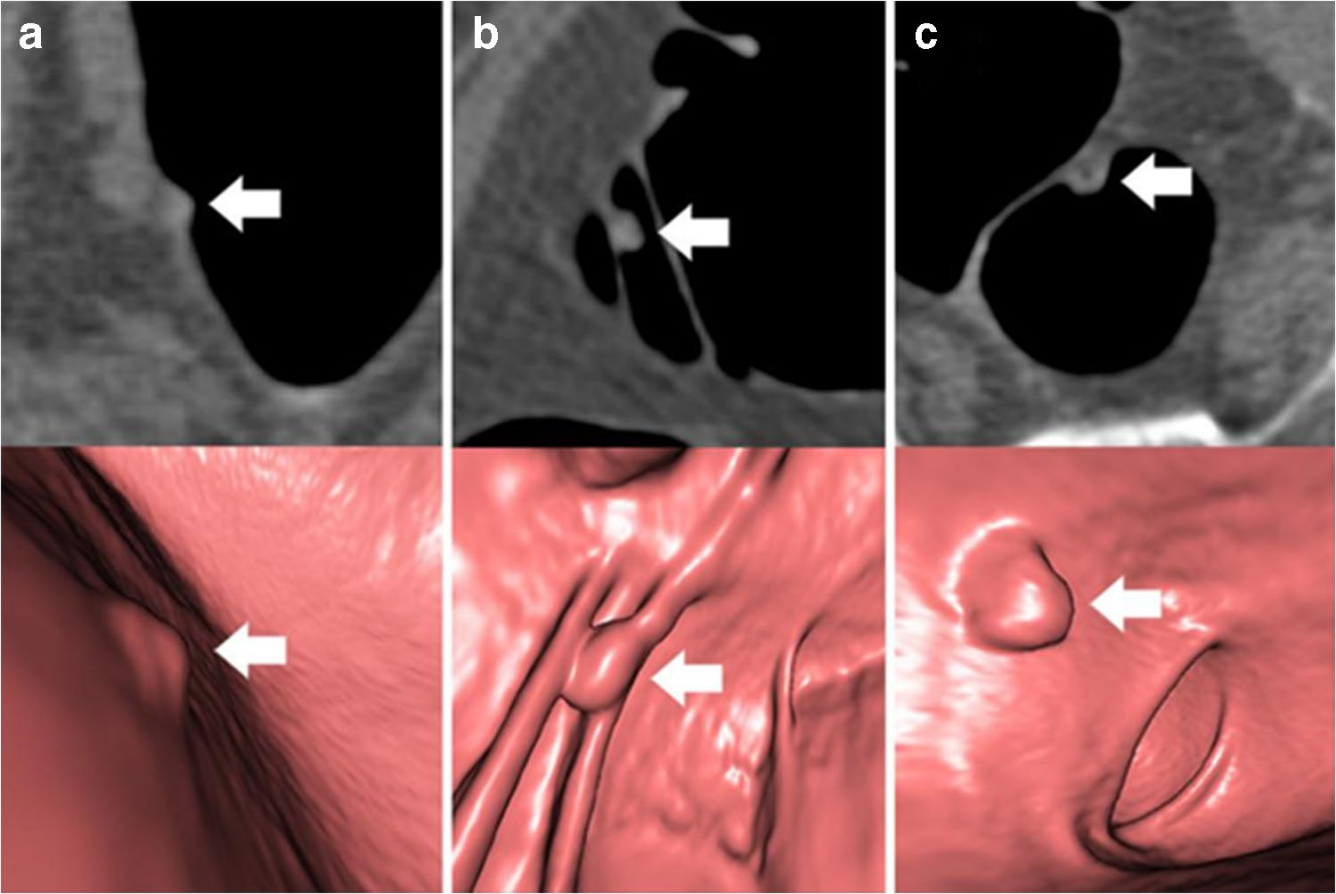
a-c Colorectal polyps of the training set (indicated by arrows) in axial 2D CT colonography images (top row) and in the corresponding virtual fly-through 3D reconstructions (bottom row). a 7-mm hyperplastic polyp in the rectum of a 58-year-old woman. b 8-mm tubular adenoma in the transverse colon of a 74-year-old woman. c 9-mm tubulovillous adenoma in the rectum of a 67-year-old man
